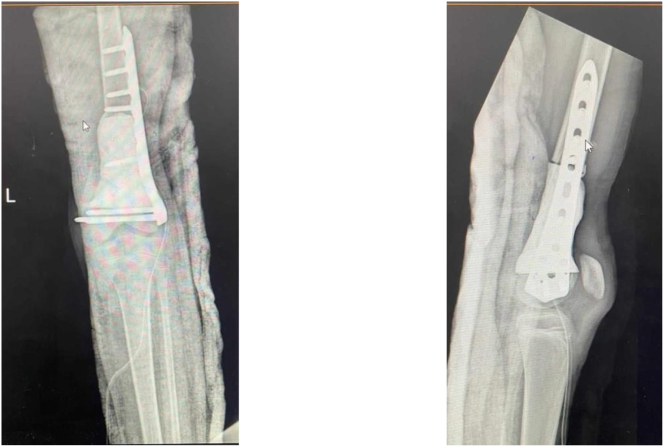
Post-operative X-ray: Bone reconstruction used spacer and stabilization using an anatomical plate.
